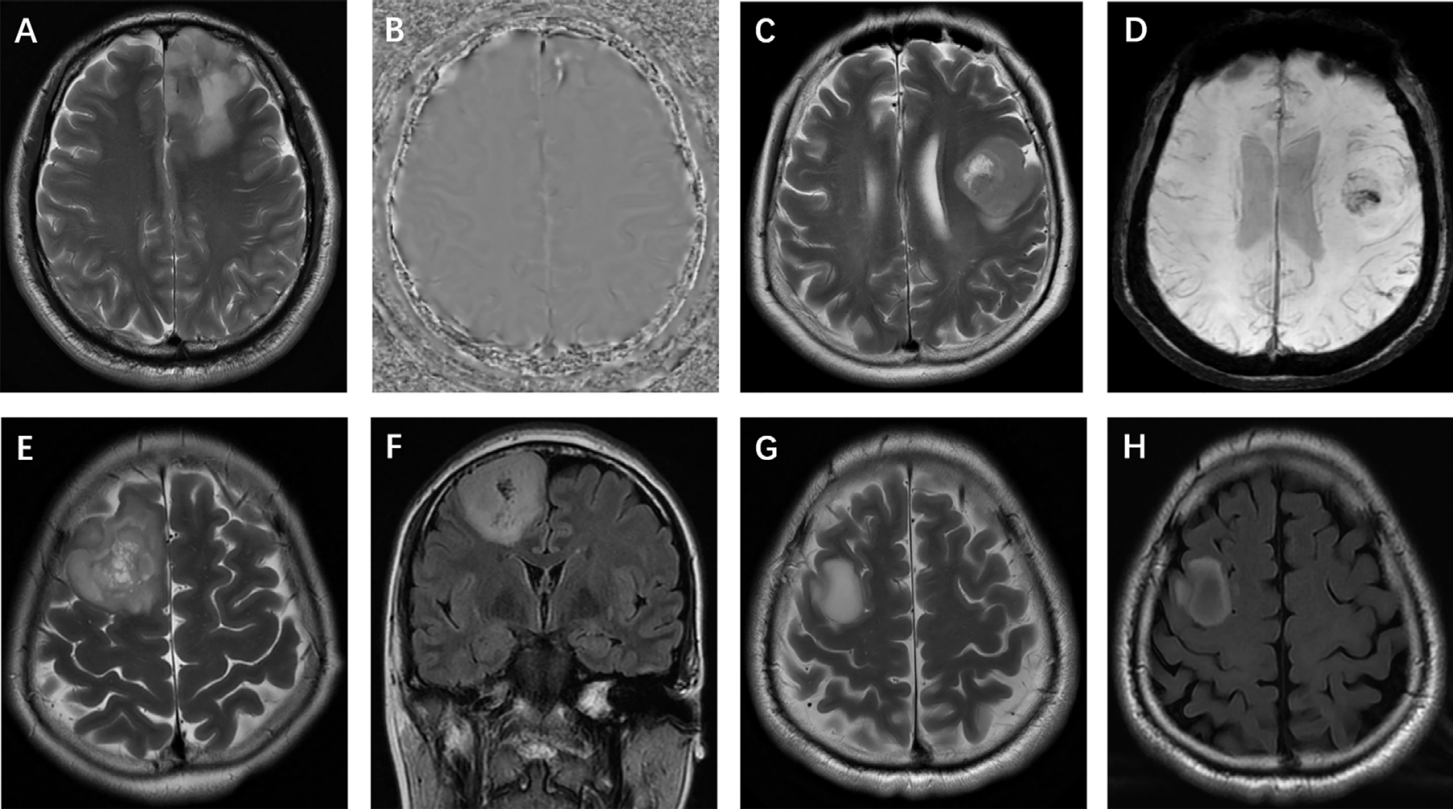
Glioma morphologic characteristics. (A, B) Calcification: T2-weighted imaging and phase image on SWI show calcification in a left frontal isocitrate dehydrogenase (IDH)-mutant oligodendroglioma; (C, D) Hemorrhage: T2-weighted imaging and SWI show hemorrhage in a left frontal IDH-wildtype glioma; (E, F) Cystic change: T2-weighted and FLAIR imaging show small cysts in a mutant-IDH oligodendroglioma; (G, H) T2-FLAIR mismatch sign: T2-weighted and FLAIR images show a T2-FLAIR mismatch sign in a mutant-IDH diffuse astrocytoma.
